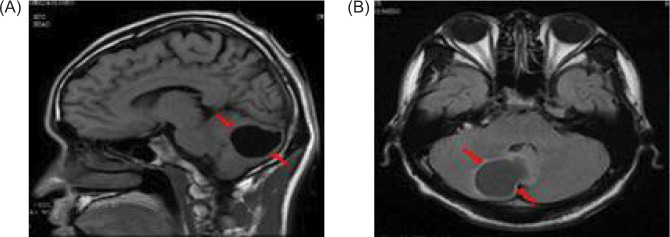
Magnetic resonance imaging (MRI) of the brain reveals a cystic mass in the right cerebellar hemisphere, approximately 40 mm in size (red arrow); (A) sagittal position, and (B) flair view.
